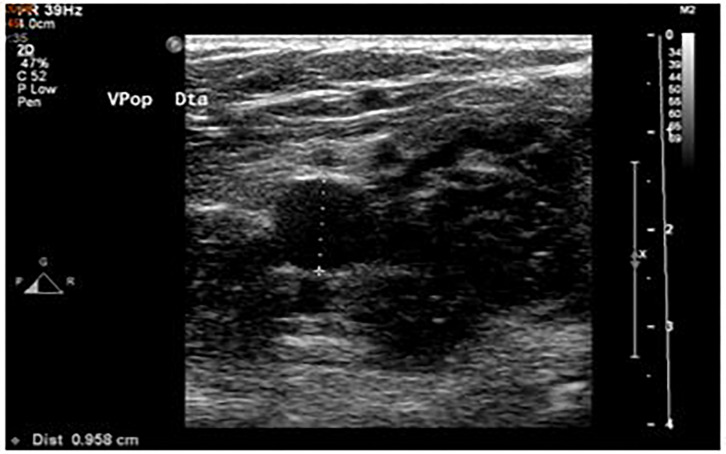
Measurement of venous diameter of right popliteal vein by lower limb venous Doppler ultrasound.
